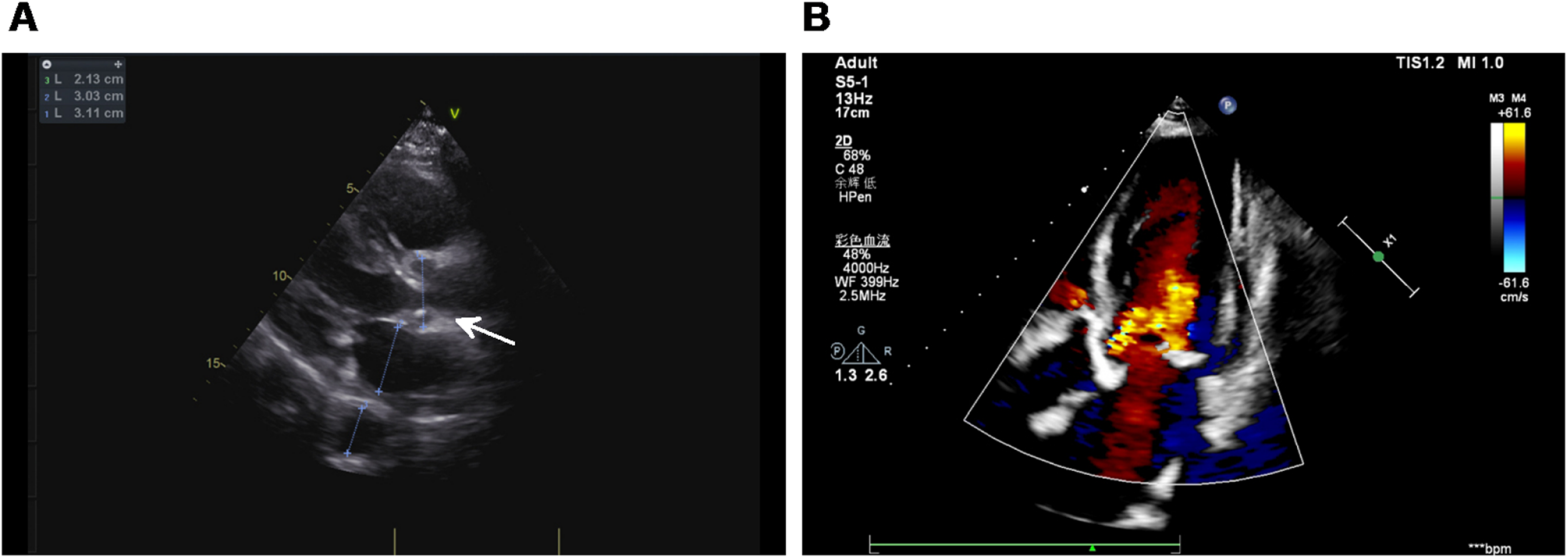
Echocardiographic assessment of aortic valve function. (A) Two-dimensional echocardiography reveals mild aortic valve stenosis and incomplete valve closure (white arrow). (B) Color Doppler imaging demonstrates turbulent flow consistent with mild-to-moderate aortic stenosis
